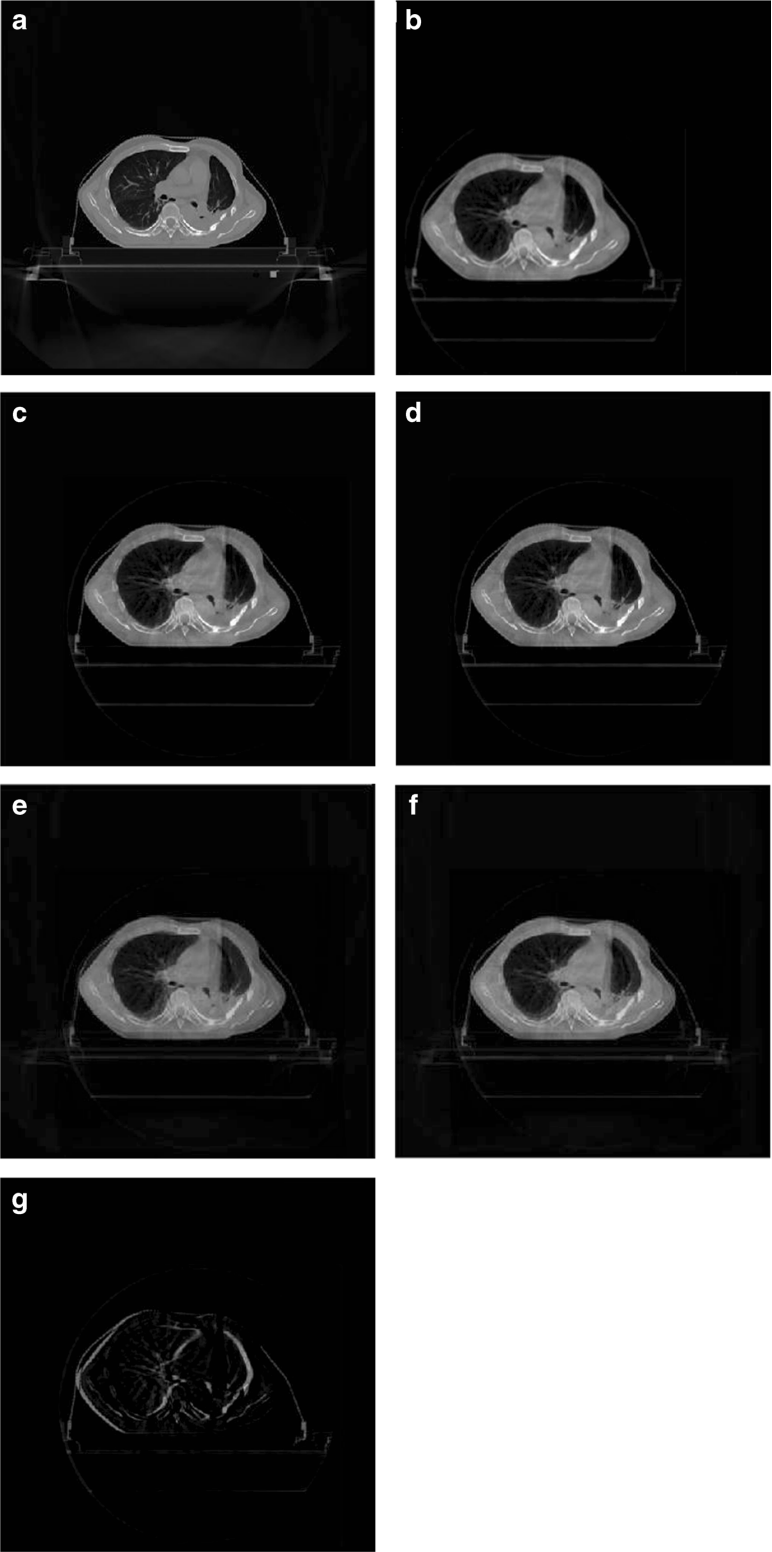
Results of registering CBCT to CT images. Part a is the original CT image; Part b is the CBCT image; Part c is the registration result by using conventional MI method; Part d is the .registration result by using our proposed method; Part e is the fusion of CBCT and CT images with conventional MI method; Part f is the fusion of CBCT and CT images with our proposed method; Part g is the difference (f–e) of registered CBCT images by using the two methods
